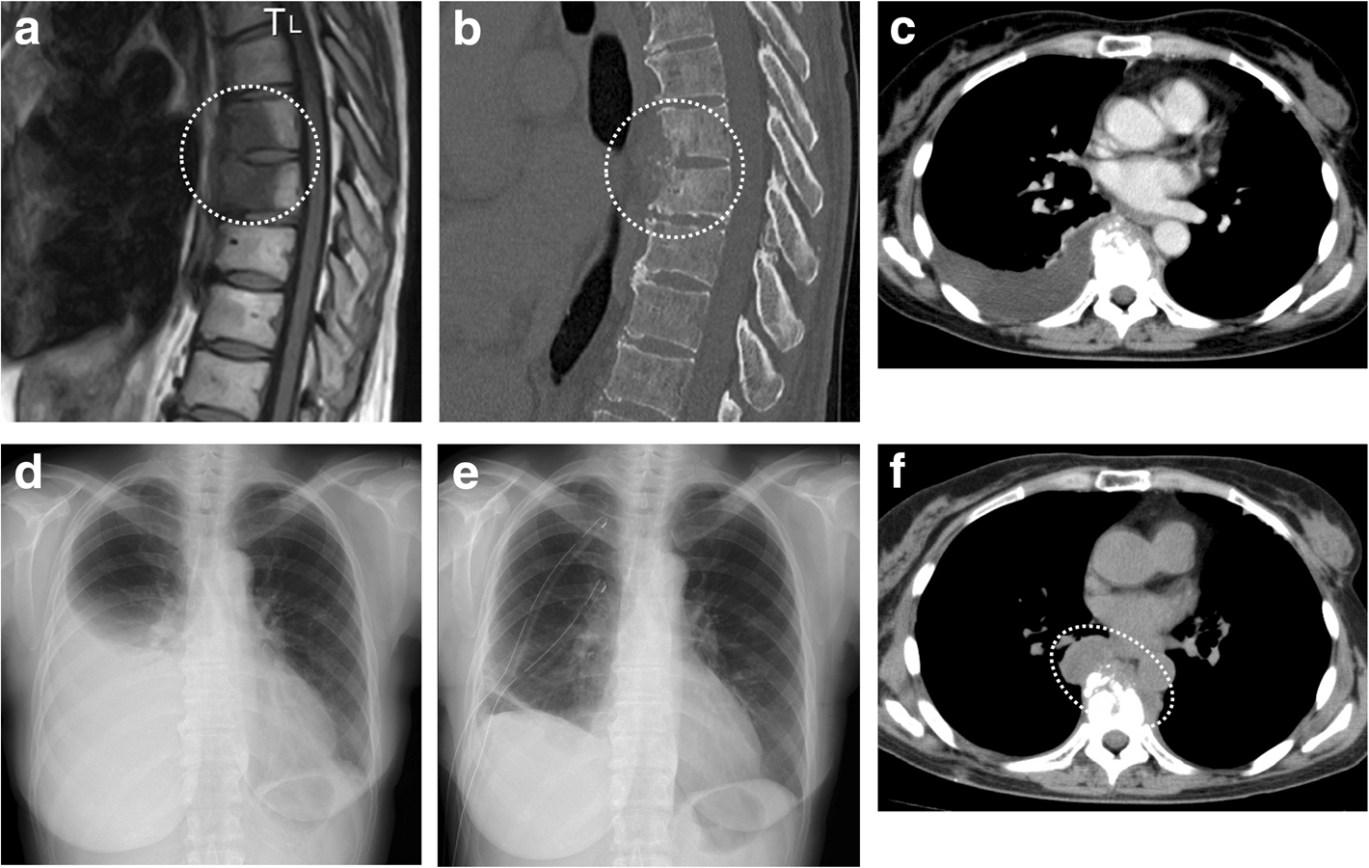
Imaging findings before antimycobacterial treatment (a T1-weighted spinal MRI obtained 2 months before admission, b spinal CT obtained 3 weeks before admission, c enhanced chest CT scan on admission, d chest X-ray film after treatment with ampicillin/sulbactam for 11 days, e chest X-ray film during chest drainage and administration of cefoperazone/sulbactam following thoracoscopic curettage, f chest CT scan one month after chest tube removal). Spinal MRI and CT detected a T7/8 vertebral lesion (circled). Chest drainage achieved satisfactory re-expansion of the right lung. After removal of the chest tube, a paravertebral lesion was detected on CT (circled)
